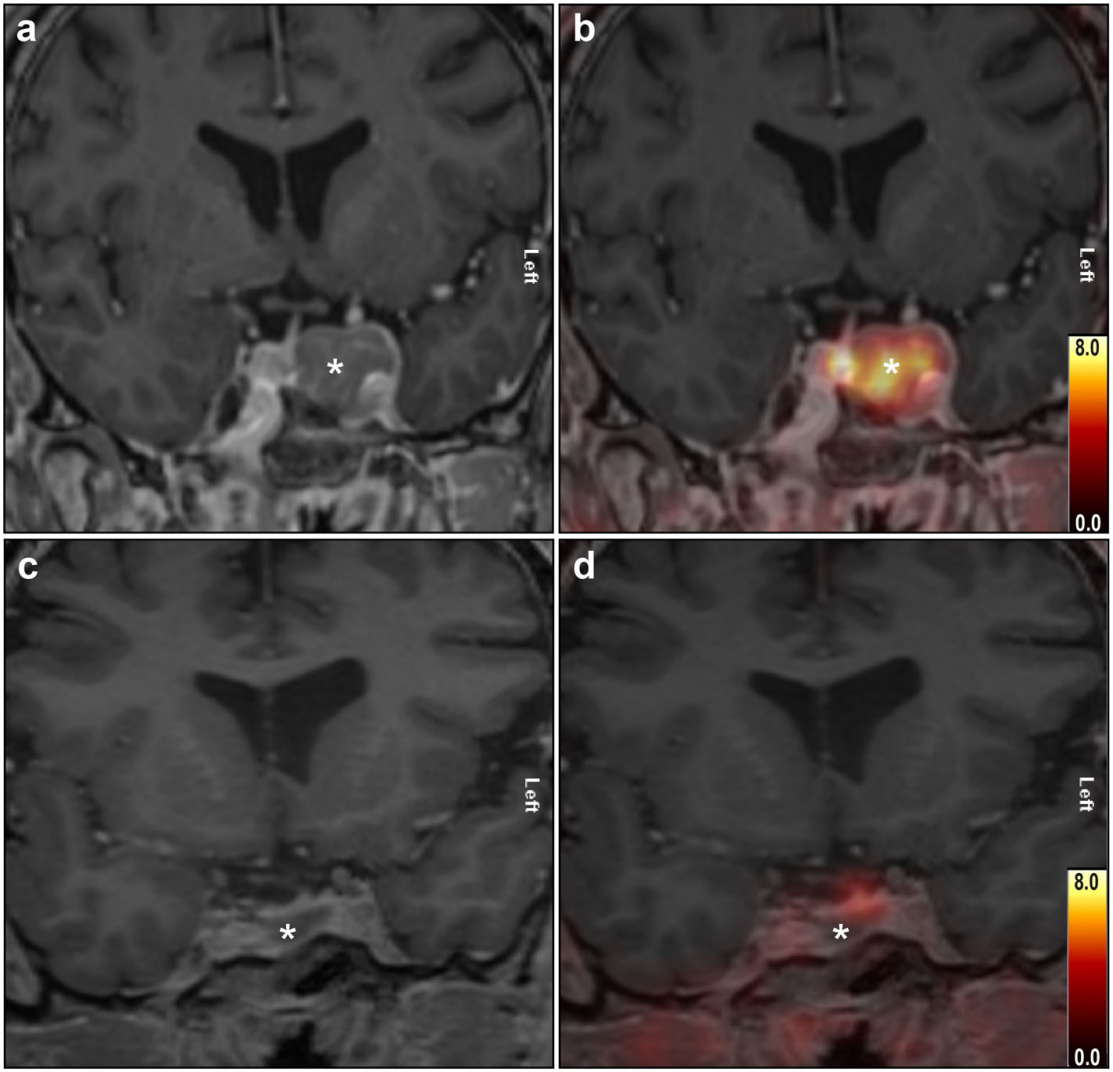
Coronal T1-weighted contrast-enhanced MRI and fused PET/MR images of a ^68^Ga-DOTATATE PET-positive (a, b) and PET-negative (c, d) NFMA. The colour bars represent SUV. a MRI shows a pituitary macroadenoma (asterisk) located laterally in the sella with an invasion of the left cavernous sinus. b After fusion with PET, intense uptake is seen in the NFMA (SUV_mean_ 5.7) as well as physiological uptake in the pituitary gland on the right side of the adenoma. c In this case, the largest part of the adenoma (asterisk) is located on the right side of the pituitary stalk. d The fused PET/MRI shows no uptake in the NFMA (SUV_mean_ 1.1) and moderate physiological uptake in the pituitary gland situated on top of the adenoma
